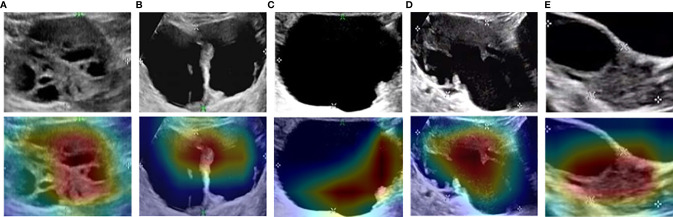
Examples of class activation mapping using the transfer learning ResNet34 model. The model correctly identified malignant (A), borderline (B), and benign (C) SOTs. Both the model and the senior sonographer made the same mistakes: borderline SOT misdiagnosed as malignant SOT (D); and malignant SOT misdiagnosed as borderline SOT (E).
